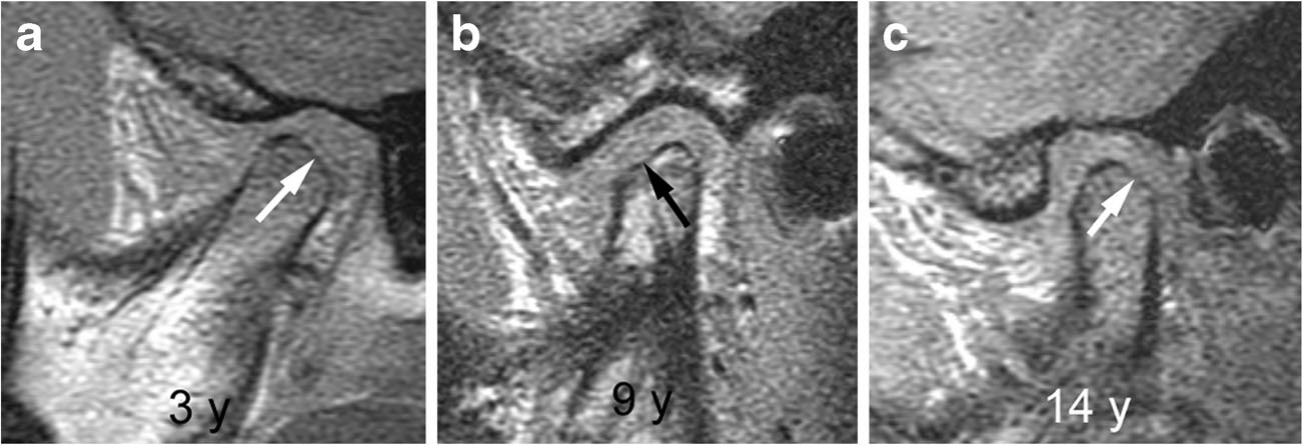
Mild condylar flattening (grade 1). a-c Sagittal oblique T1-weighted gradient echo images (TR/TE 300/4.2 ms, flip angle 80°, without fat saturation) from different children obtained at 3 years (a), 9 years (b) and 14 years (c) show flattening involving part of the condylar surface (arrows)
